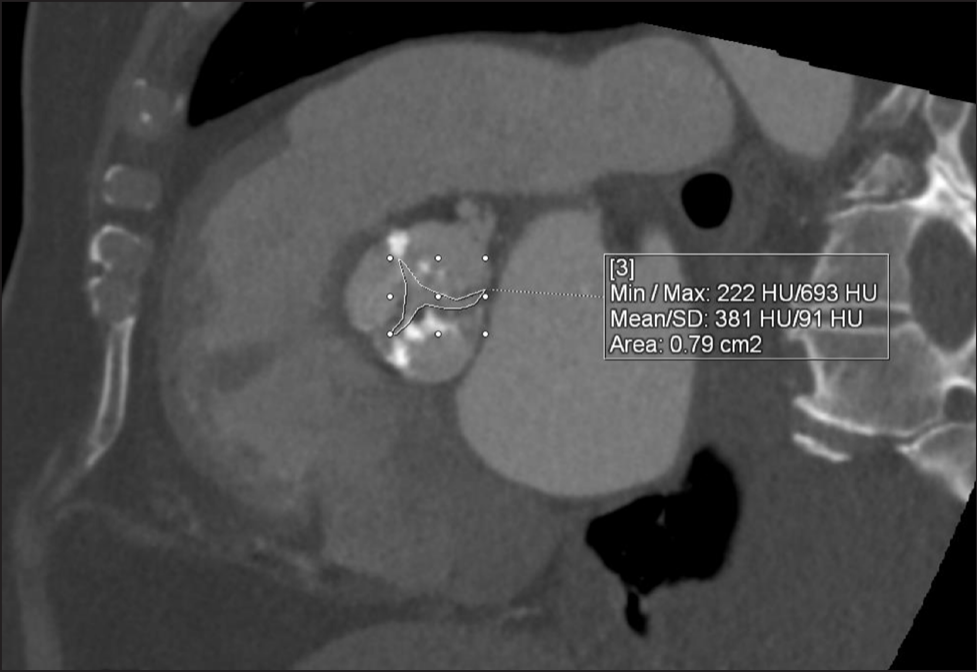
Cardiac computed tomography showing severe calcific aortic valve stenosis (valve area 0.79 cm^2^) via planimetry.
